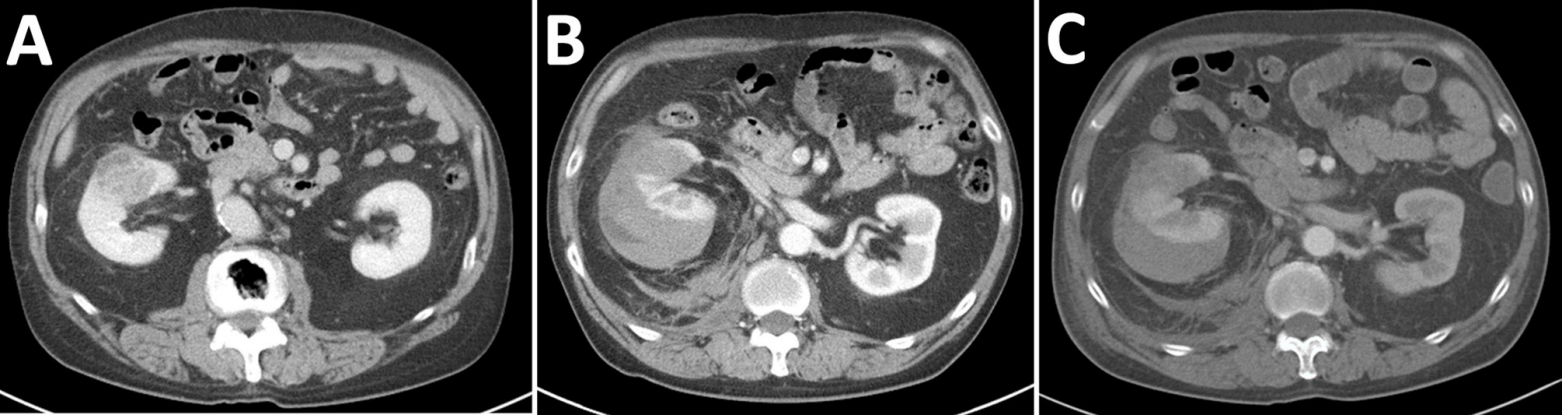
CT scans in a case of fatal renal abscess caused by *Porphyromonas gingivalis* and subcapsular hemorrhage, Japan. A) Contrast enhanced CT 3 days before patient admission shows a 3.5-cm large right renal mass, which was suspected to be renal carcinoma based on imaging findings. B) Contrast enhanced CT at admission. The patient was hospitalized for bleeding from the renal mass. C) Contrast enhanced CT 1 day after admission, showing a shrinking hematoma. CT, computed tomography.
